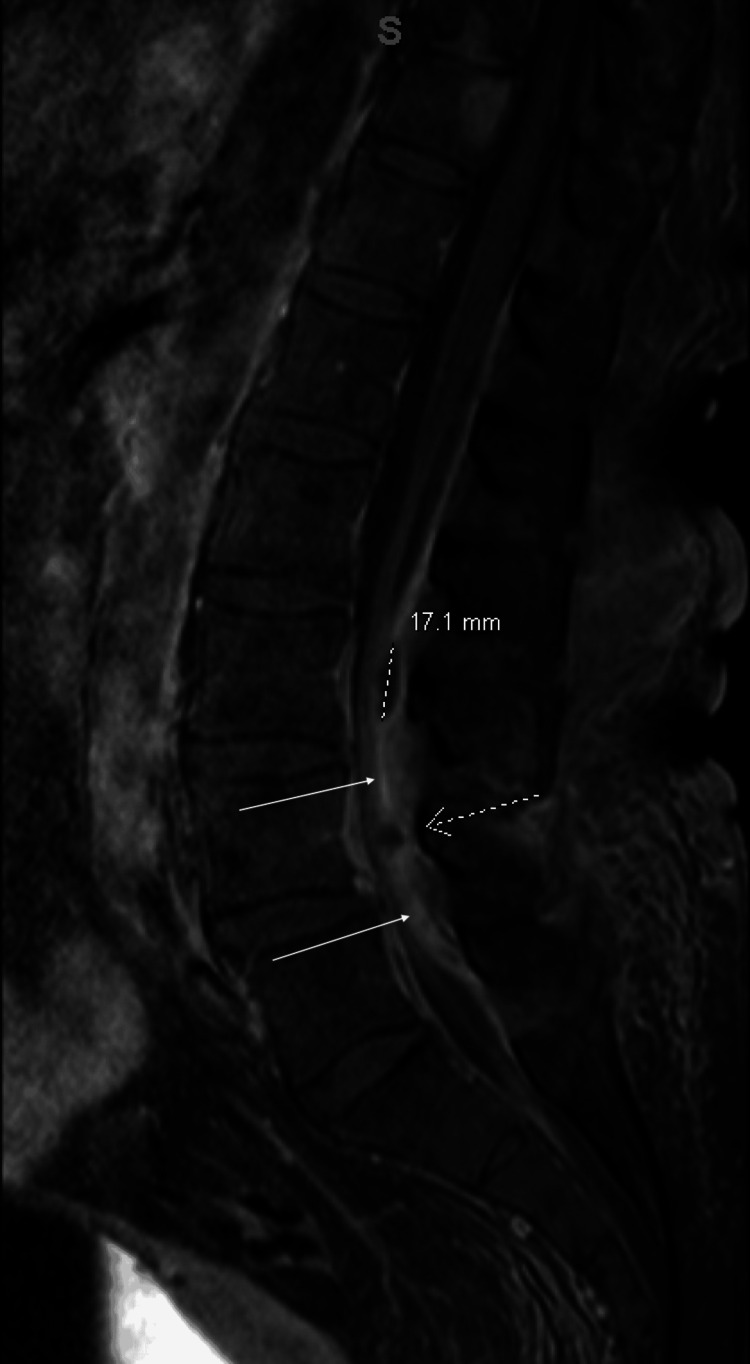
Sagittal short tau inversion recovery (STIR) MRI of the lumbar spine with contrast Key neurological exam findings: 3/5 strength and +1 patellar reflexes in bilateral lower extremities; no saddle anesthesia, Hoffmann sign, dysdiadochokinesia, or clonus. 17.1 mm: At the L3 level, there is enhancement and thickening of the dura, as well as a spinal epidural abscess measuring 17.1 mm in the cranio-caudad dimension. Dashed arrow: At the L4 level, there is a smaller fluid pocket noted within the posterior aspect of the epidural space. Solid white arrows: The enhanced dura is thickened and inflamed, causing clumping of the nerve roots and contributing to severe central stenosis.
